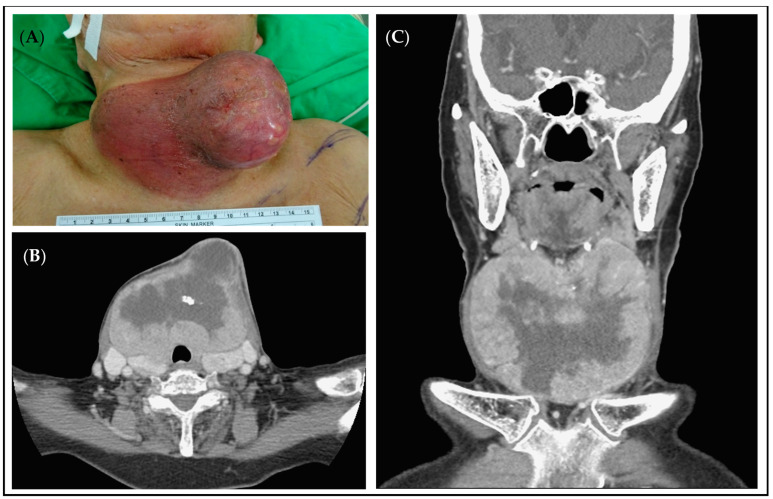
(A): A 92-year-old woman suffered from a large thyroid tumor with skin ulceration and cellulitis. (B,C): The neck CT scan showed an enhancing necrotic mass 10 × 11 × 10 cm with calcification in lower neck submental space, abutting bilateral thyroid glands.
